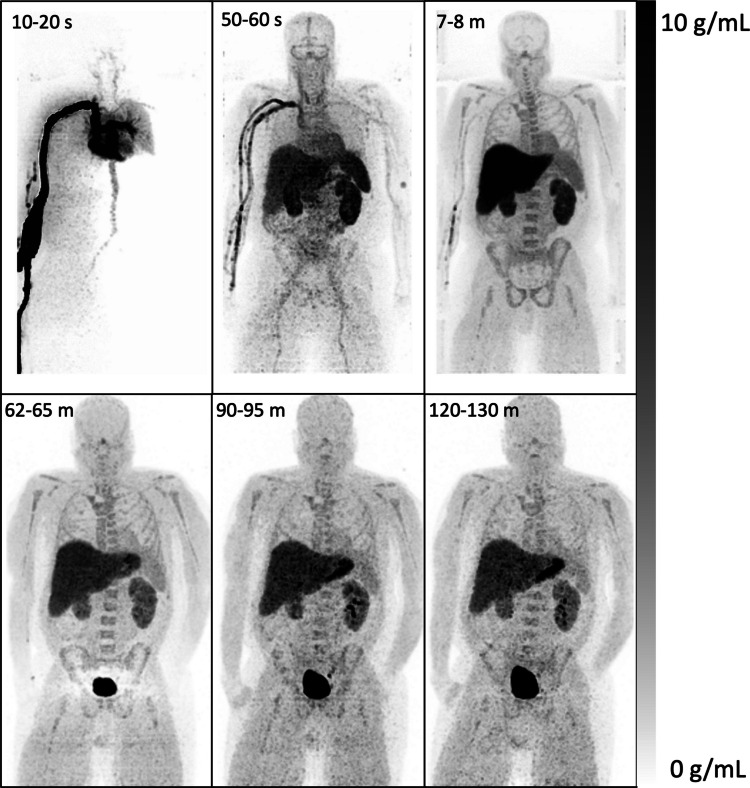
Dynamic PET maximum-intensity pixel images of [^11^C]TMP in a 52 year old male without active infection. Images were acquired on a whole-body PET/CT scanner following injection of 503 MBq of [^11^C]TMP. Image acquisition times post-injection are listed in the upper left corner
